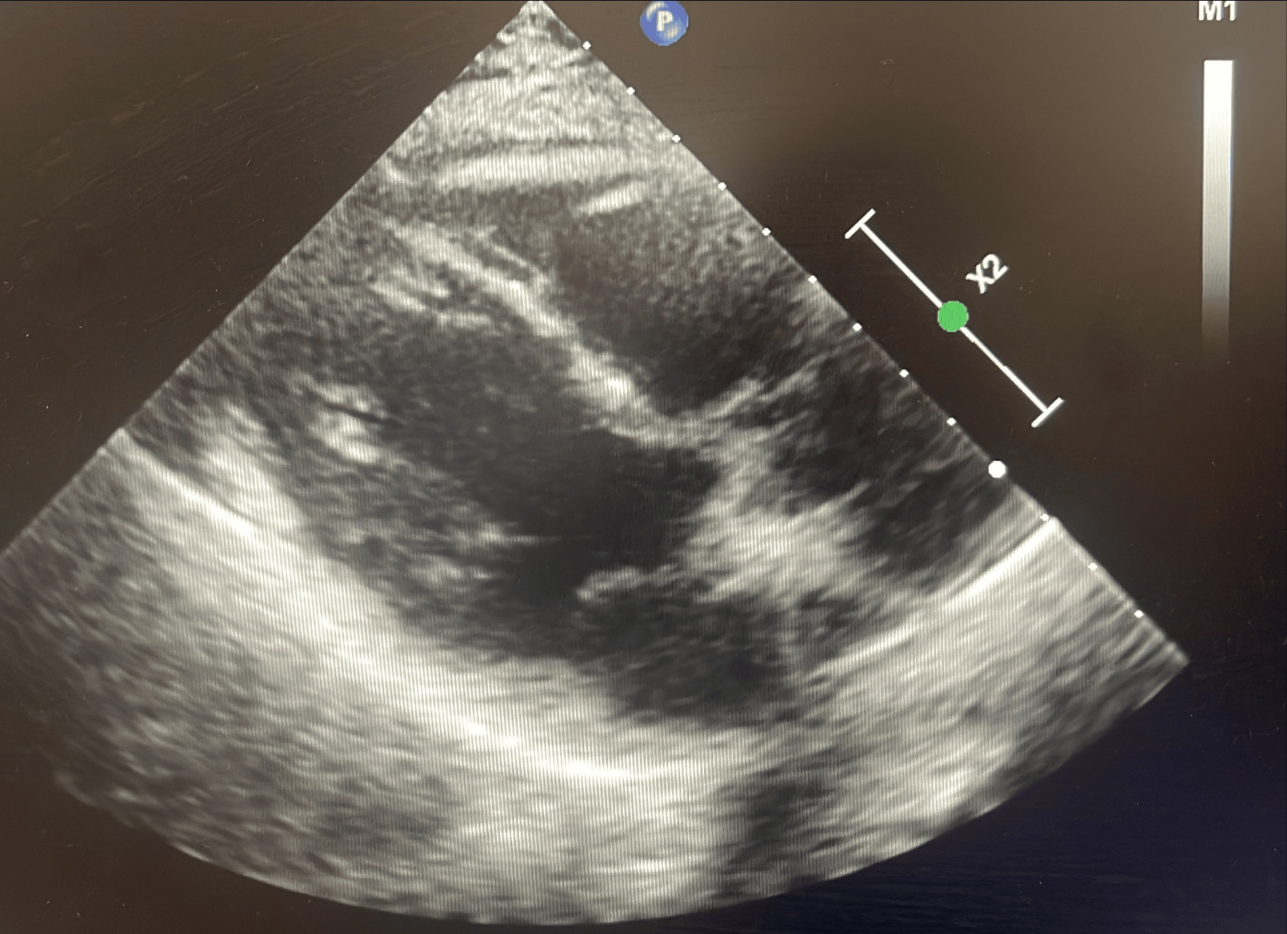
Transthoracic echocardiogram (TTE) showing normal biventricular systolic function with an estimated LVEF of 60–65%. Concentric left ventricular remodelling is observed. No valvular abnormalities or aortic root dilatation are present. The TTE was performed to rule out cardiac involvement, confirming that the disease process was confined to the large vessels.
